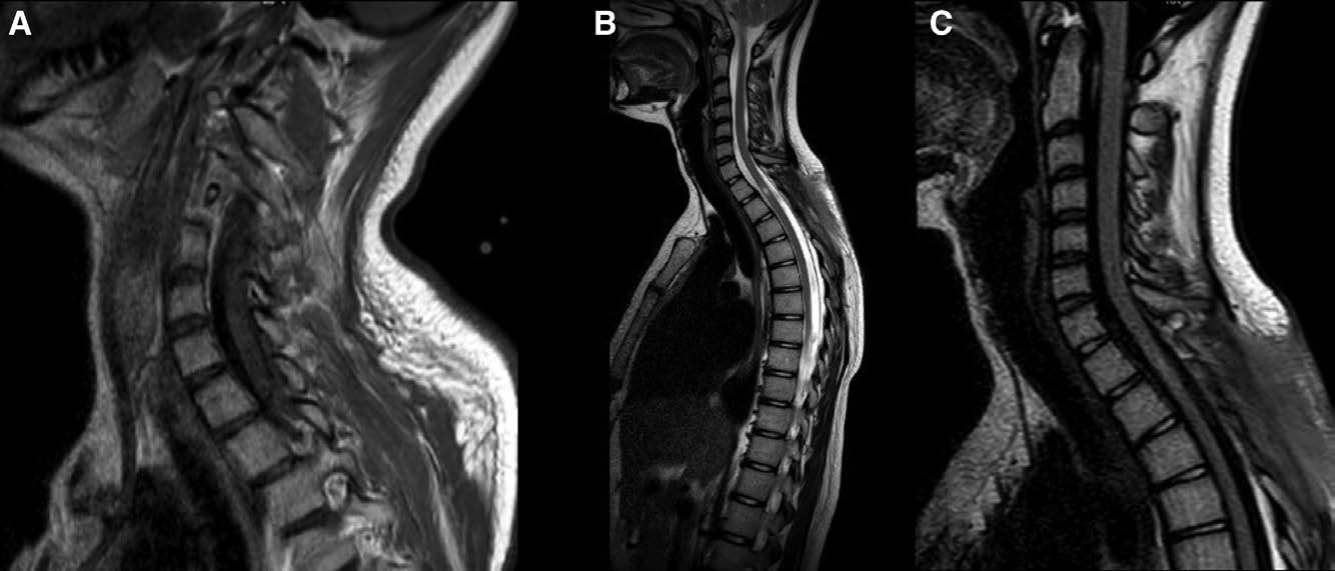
MRI follows up; (A) sagittal T1WI; 24 hours after surgery; shows no mass effect with a drain. (B) Sagittal T2WI and flair (C); 2 months after discharge; show no recurrence of the tumor with a free voiding of CSF. MRI = magnetic resonance imaging.
